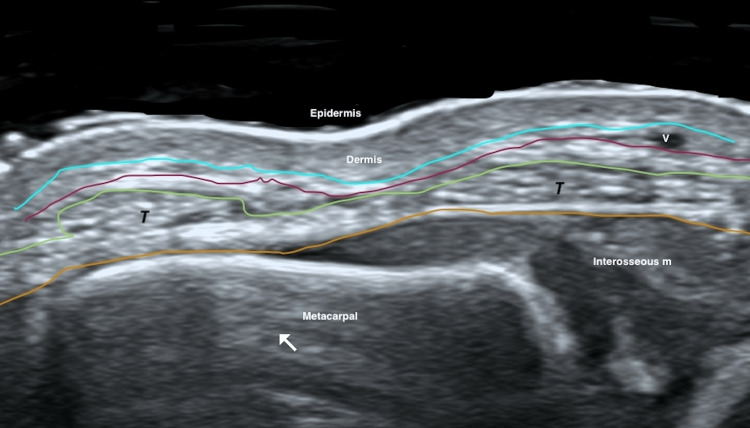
HRUD of normal dorsal hand anatomy (transverse view) Color overlays delineate key fascial planes and structures: aquamarine line, superficial dermal fascia; purple line, superficial venous fascia; green line, intermediate dorsal fascia; yellow line, deep dorsal fascia. The superficial venous plexus (v), extensor tendons (T), and metacarpal bone (white arrow) are indicated. Abbreviations: HRUD, high-resolution ultrasound with Doppler; v, superficial venous plexus; T, extensor tendons.
